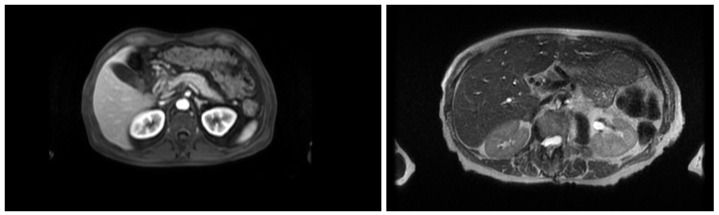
The (left) picture shows a T1-weighted MRI scan, where fat-rich tissues appear hyperintense, providing clear anatomical boundary definition. The (right) picture shows T2-weighted MRI scan, where fluid-rich structures such as cerebrospinal fluid appear hyperintense, enhancing contrast in edema, inflammation, and other fluid-related pathologies.
